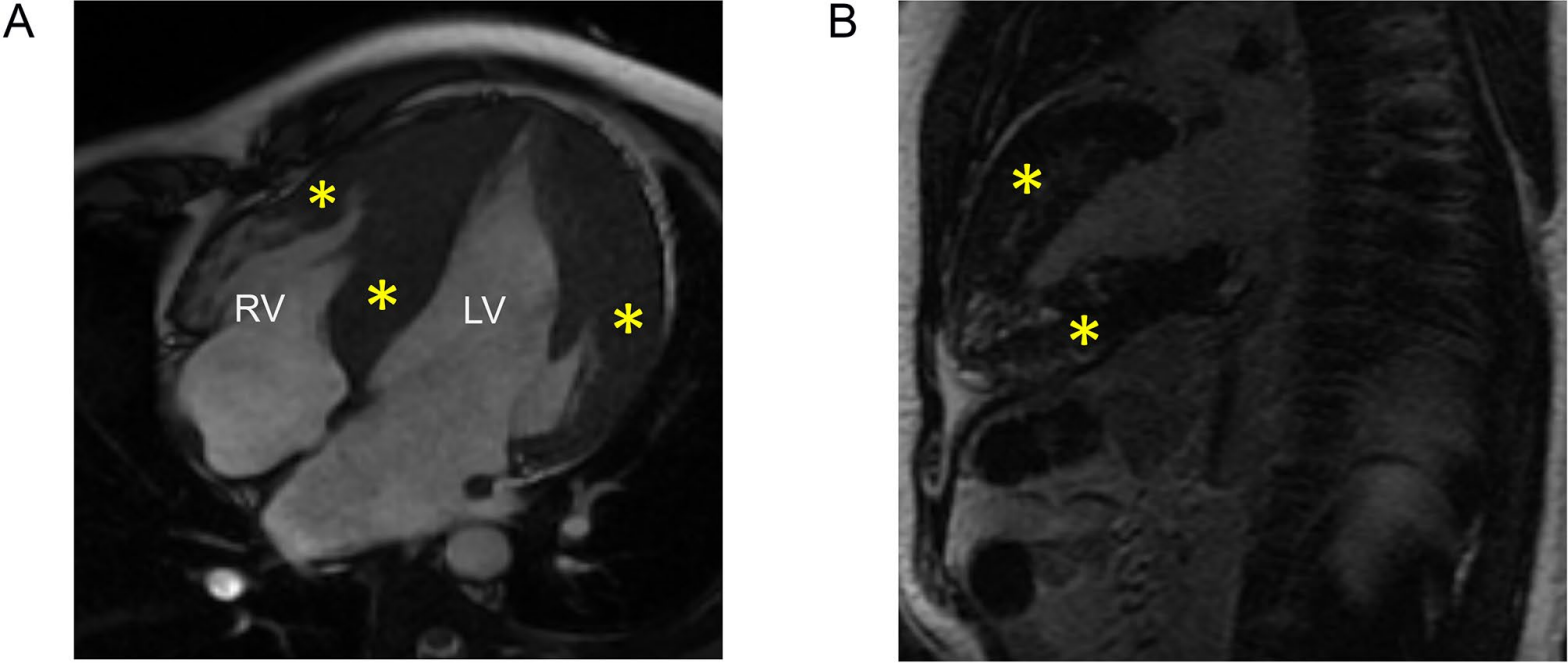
Cardiac MRI (SSFP Cine sequences) of patient CIII-5 showing marked hypertrophy in the region of the interventricular septum and lateral wall of the left ventricle (yellow asterisks) along with right ventricular free wall hypertrophy (red asterisk) in the apical four-chamber view (A). The two-chamber long-axis view shows patchy areas of mid-wall left ventricular late gadolinium enhancement in the anterior and inferior walls (yellow asterisks) (B).
